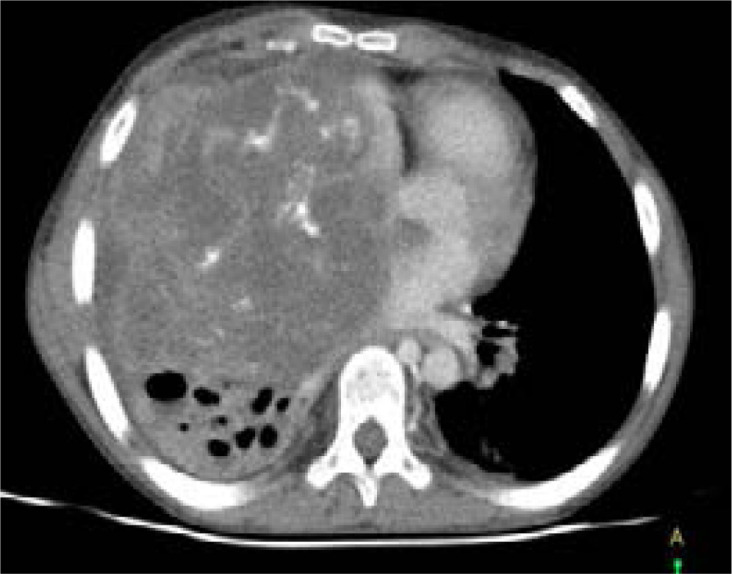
Contrasted axial chest CT image showing an extensive hetero-complex mass in the right hemi-thorax with pericardial invasion
